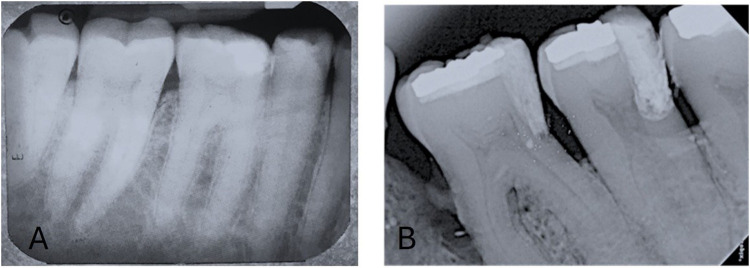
Postop IOPA radiographic representation of vital pulp therapy with the use of bioceramic materials. (A) Indirect pulp capping with Biodentine and, (B) Direct Pulp capping with MTA.
